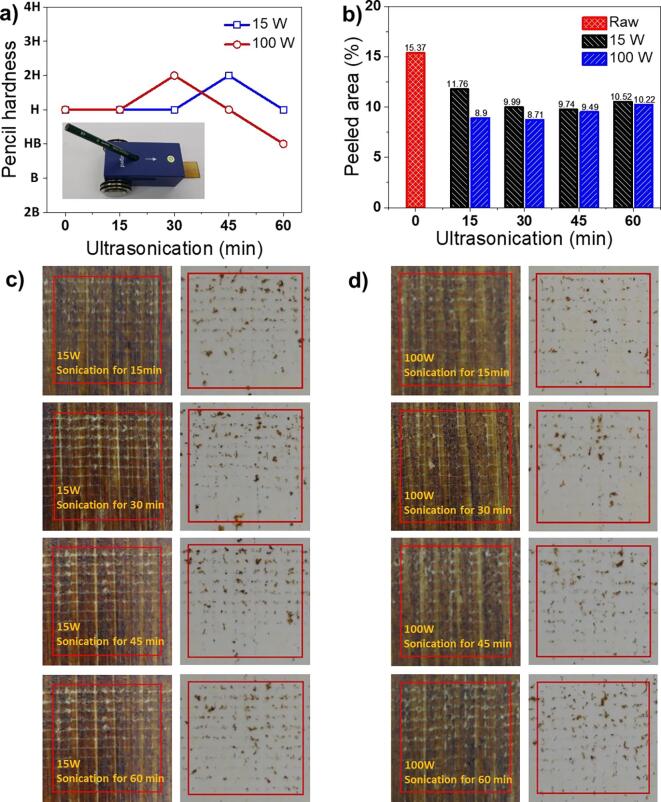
a) Pencil hardness and b–d) adhesion measurements of thin films prepared with raw and ultrasonicated lacquer sap solutions.
